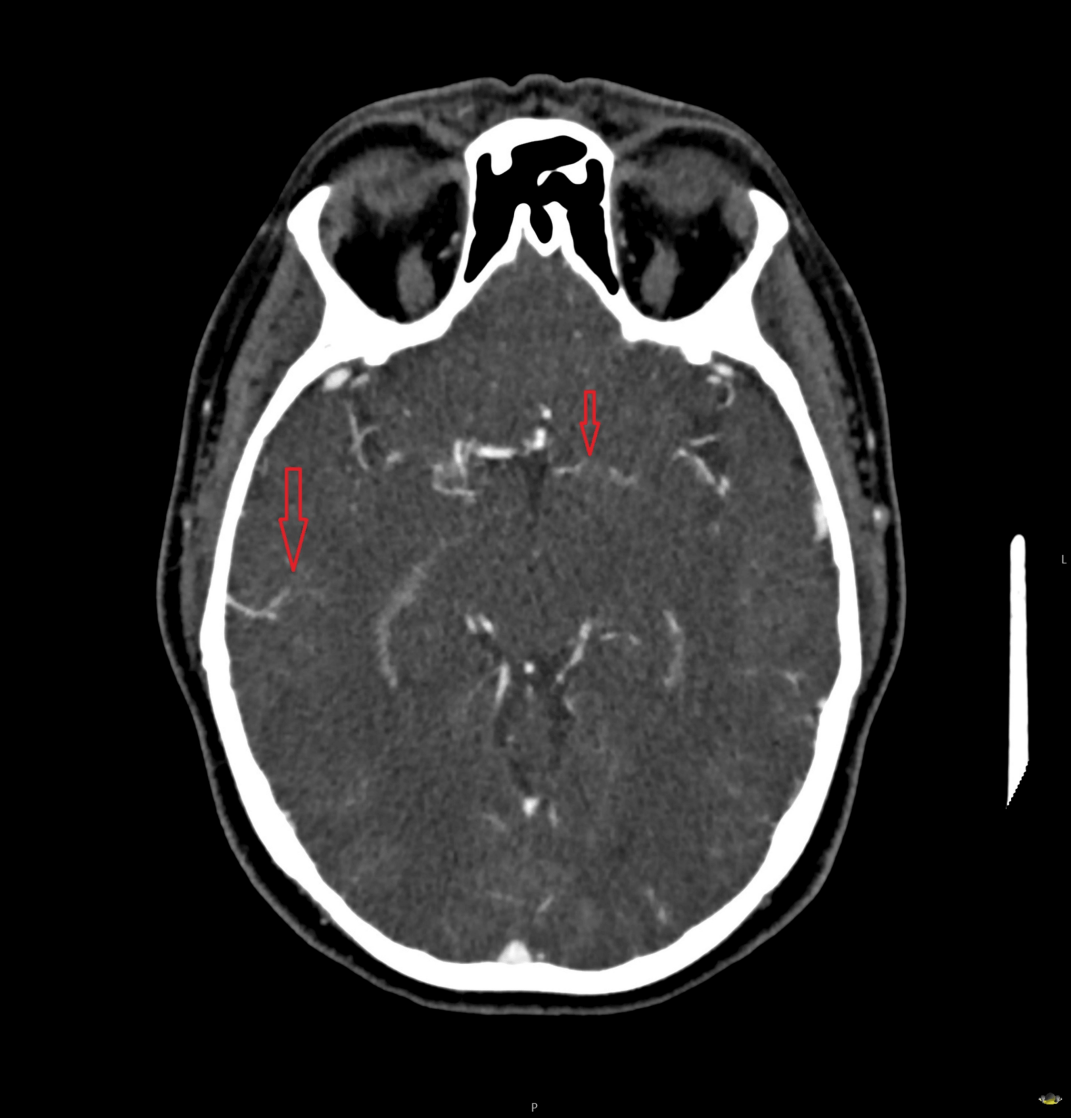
CT imaging showing early signs of Moyamoya disease with collateral vessel formations
